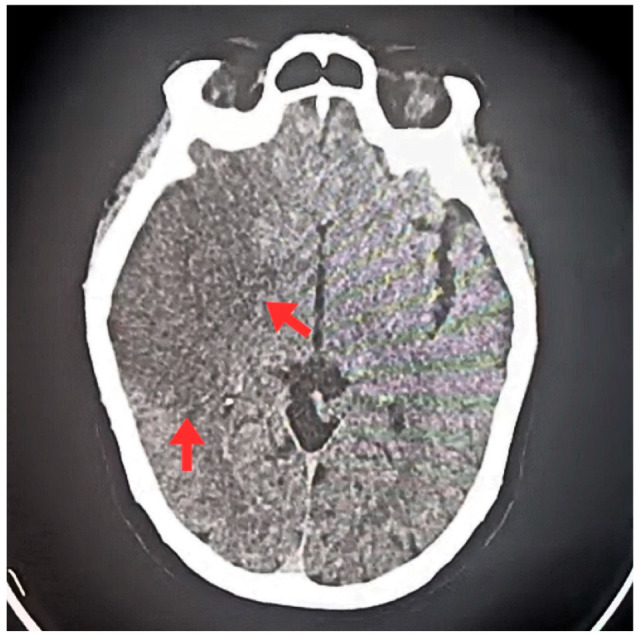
Non-contrast computed tomography of the brain at admission. The scan shows findings compatible with an ischemic stroke in the right frontoparietal region (red arrows), correlating with the patient’s predominantly left-sided neurological symptoms and consistent with contralateral lateralization of the motor deficit.
